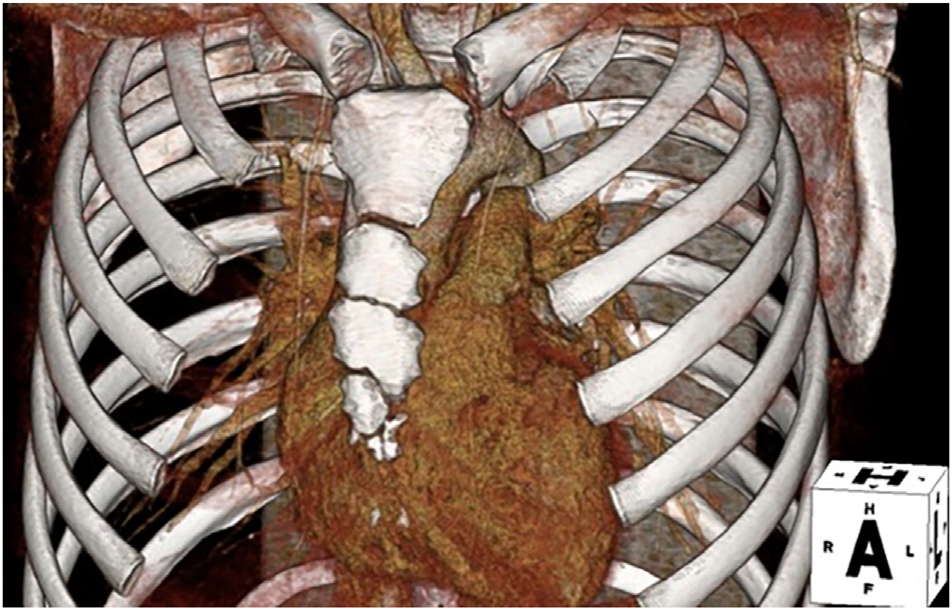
Preoperative 3-dimensional computed tomography image.
